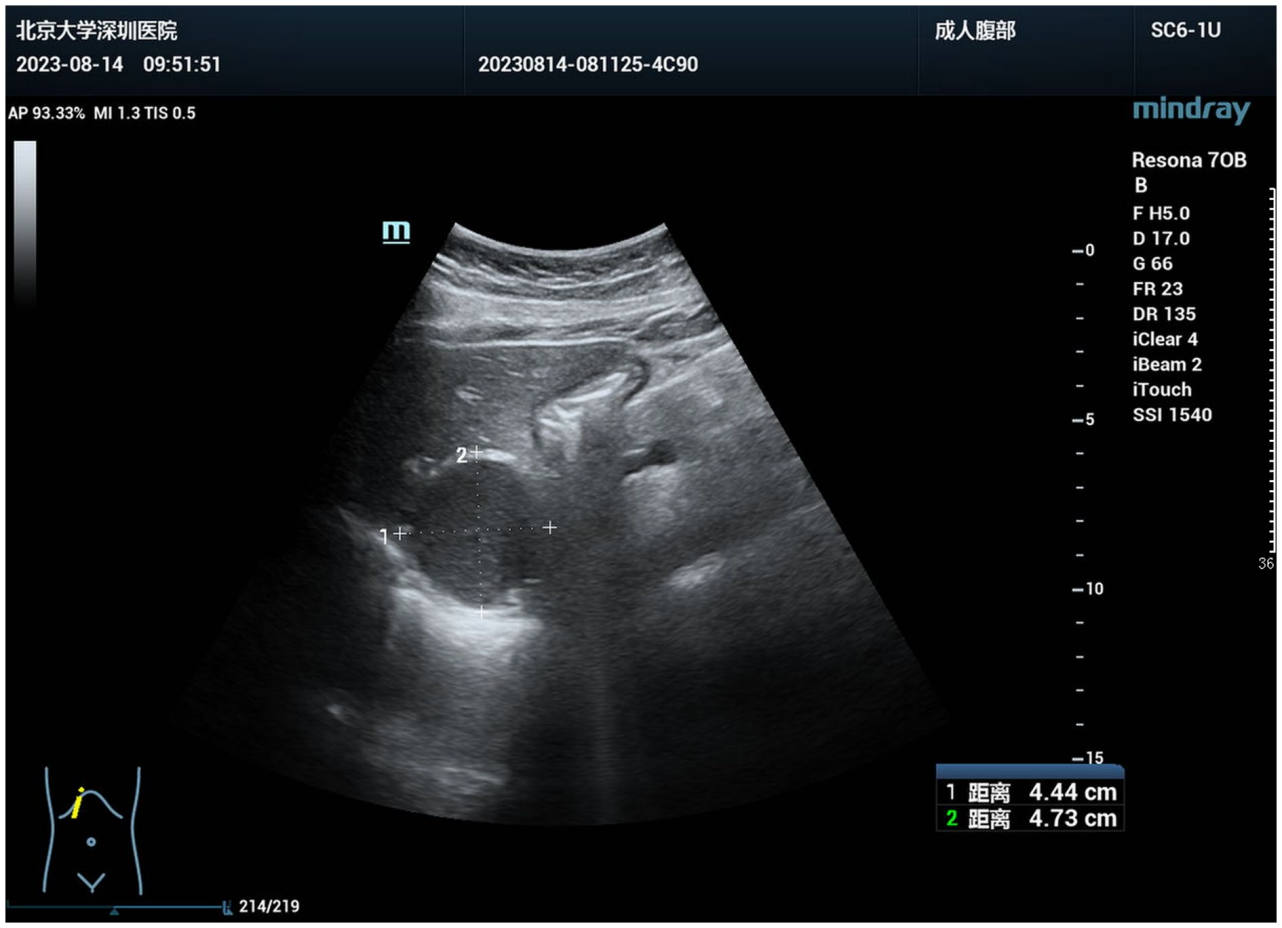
Preoperative color Doppler ultrasound revealed an anechoic mass in the hepatogastric space. The mass was well-defined, quasi-circular, with enhanced posterior echoes and poor internal echogenicity.
